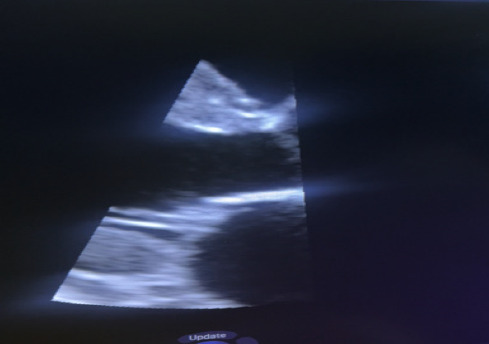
2D echocardiographic view of mitral valve demonstrating thickened mitral valve with no vegetation.
